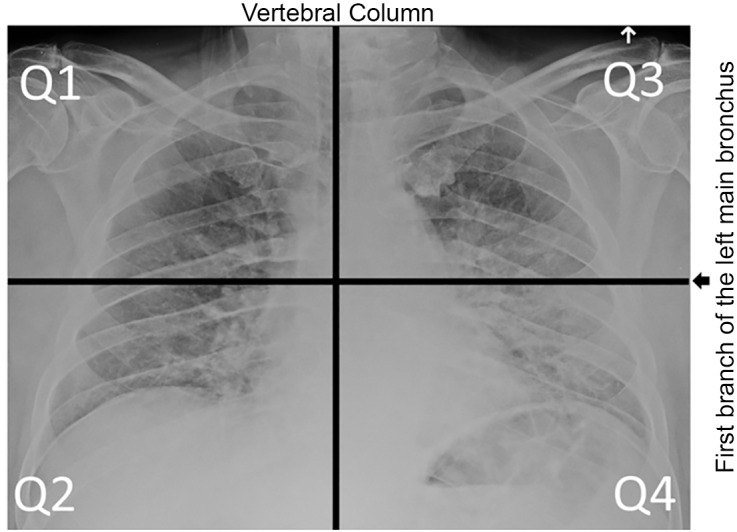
An example of radiograph assessment of lung edema (RALE) scoring in a 71-year-old man with COVID-19 who presented 5 days after symptom onset, with fever, cough and fatigue. RALE scoring: reader 1: 11, reader 2: 12. Adapted from Warren et al, 2018.^14^
